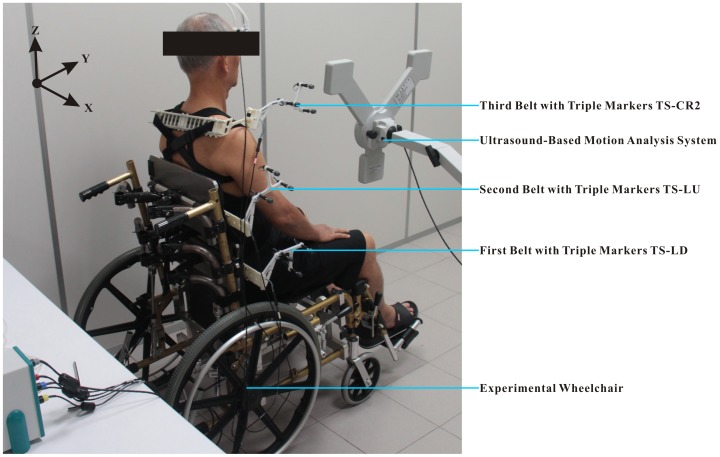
Experimental setup. The picture shows the experimental setup with experimental wheelchair, ultrasound-based motion analysis system, and miniature ultrasonic modules. The first belt with triple markers TS-LD was situated around the level of the posterior superior iliac spines and the anterior superior iliac spines. The second belt with triple markers TS-LU was placed firmly around the T12 level. The third belt with triple markers TS-CR2 was placed firmly around the T1 level.
